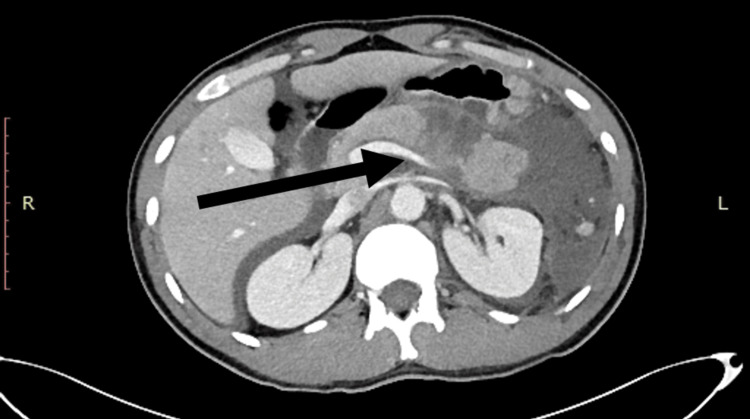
Contrast-enhanced CT scan (portal view) The black arrow indicates a full transection of the distal part of the pancreas. CT: computed tomography
